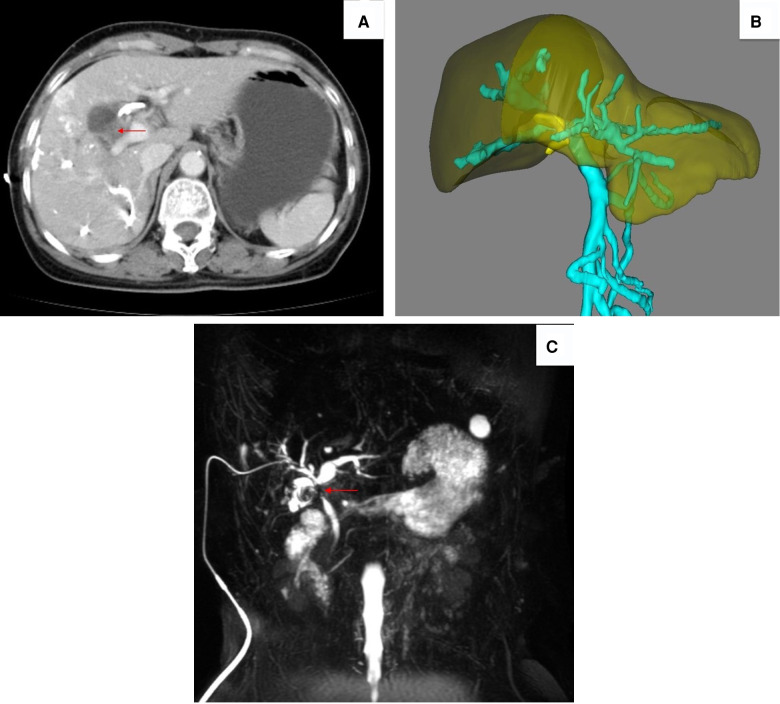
(A) Abdominal CT 1 month later revealing an improvement in hepatic duct dilation (the red arrow designates the tumor). (B) Residual liver volume (RLV) increased up to 55.57%. (C) MRCP indicating a space-occupying lesion (red arrow) at the porta hepatitis, involving the cystic duct.
